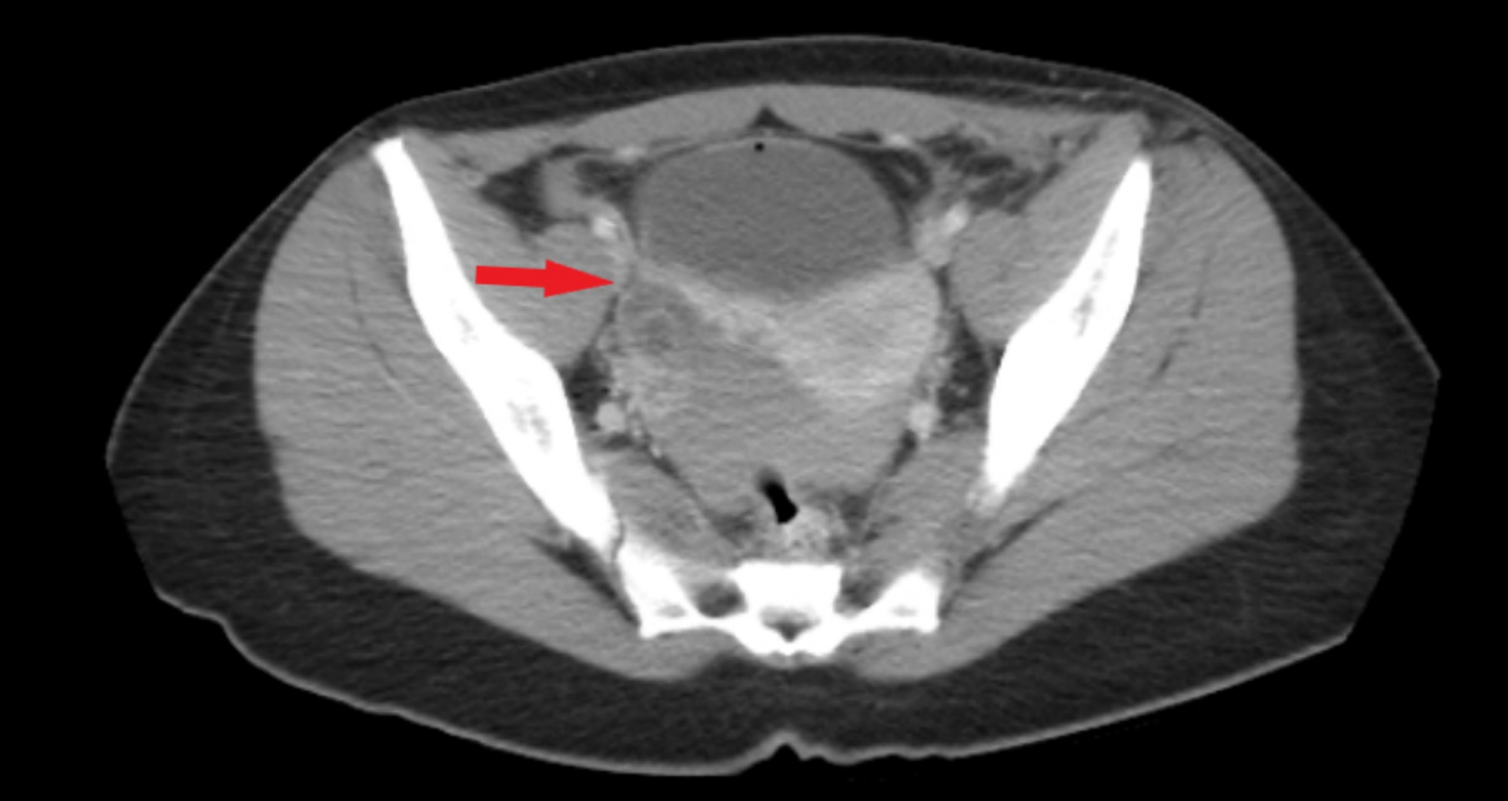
CT scan demonstrating complex, cystic mass of the right adnexa (red arrow) with pooling of free fluid in the posterior cul-de-sac.
